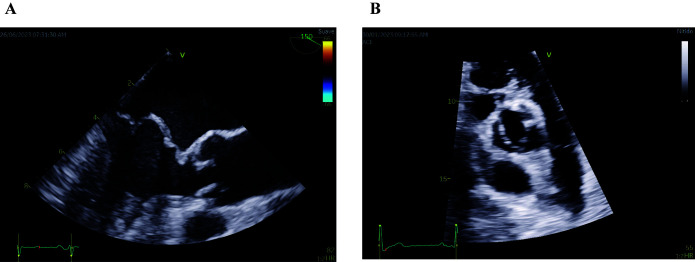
Echocardiographic images of mitral valve prolapse of both
